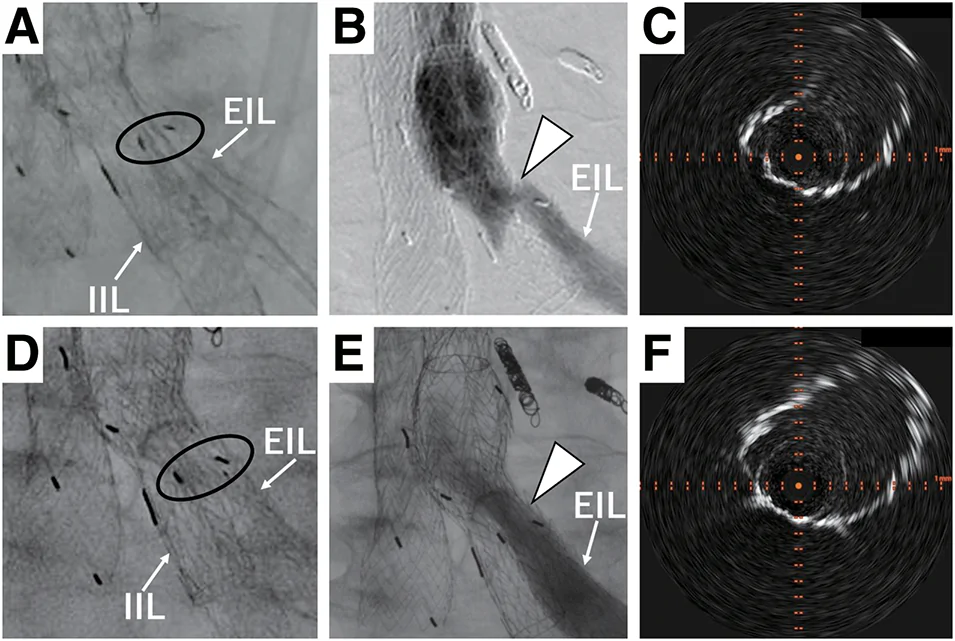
Angiographic and IVUS findings at reintervention. Angiography at reintervention demonstrates stenosis at the orifice of the external iliac limb caused by compression from the internal iliac limb (A, circled area; B arrowhead). IVUS reveals flattening and narrowing of the orifice (C). After stent deployment, angiography demonstrates resolution of the stenosis (D, circled area) and restoration of blood flow (E, arrowhead). IVUS confirms adequate stent expansion (F). EIL, external iliac limb; IIL, internal iliac limb; IVUS, intravascular ultrasound
